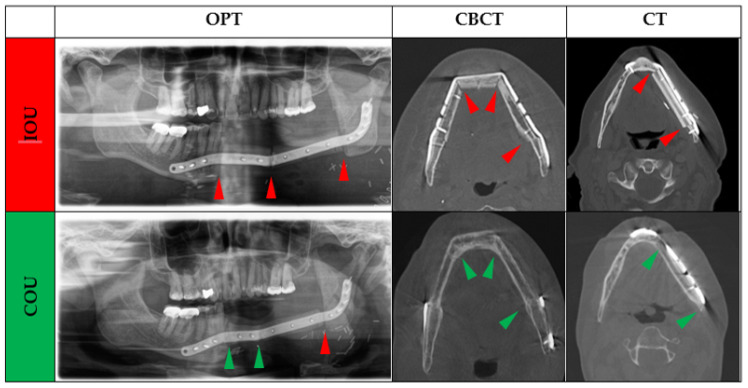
All available radiographs (OPT, CT, and CBCT-scans) were analyzed independently for ossification of each gap by the two authors. In OPT, ossification was defined as incomplete (IOU) if the interosseous gap appeared less than 50% ossified or as complete (COU) if it appeared more than 50% ossified by visual assessment. In axial CBCT or CT imaging, IOU was assigned if the two segments didn’t show fusion of the callus, marrow, or cortices, if there was a persistent gap between segments, or partial bridging between adjacent bone cortices, or marrow was noted. If the matching cortices were linked without major gaps, COU was given. Note: Green arrow: complete osseous union (COU); Red arrow: incomplete osseous union (IOU).
